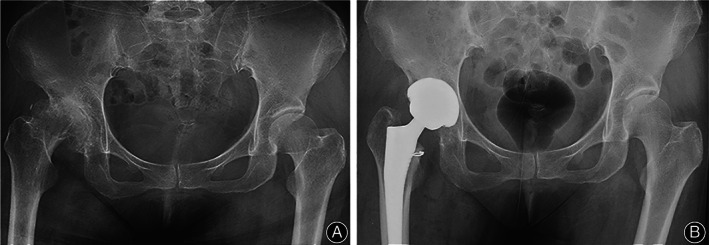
Radiographic image of a 52‐year‐old female patient. (A) Preoperative diagnosis of Crowe II hip dysplasia. (B) Measurements showed a right hip center with a height of 26.4 mm and no acetabular cup loosening or osteolysis on radiographic evaluation after 8.5 years.
